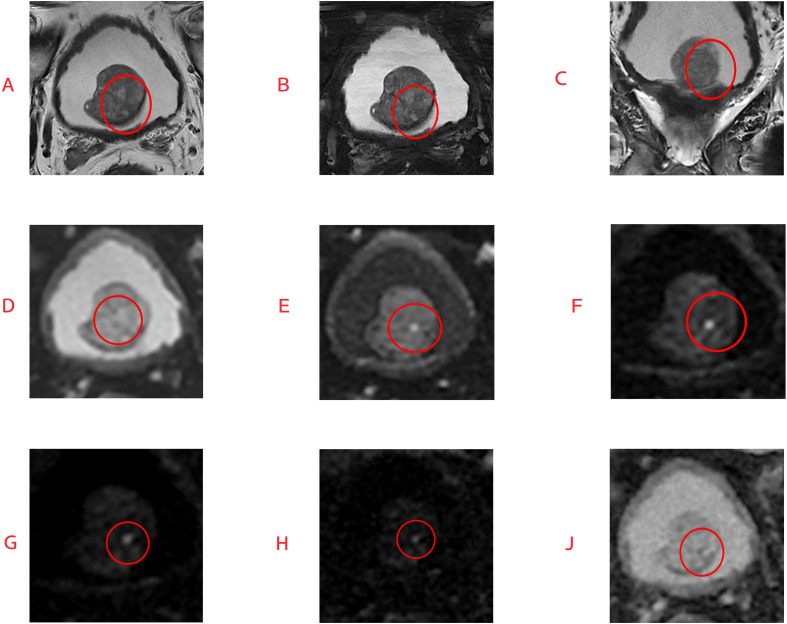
The patient is a 59-year-old male. Prostate MRI images reveal: blurred lesion margins on T2-weighted images (A–C); DWI sequences at b=0 (D) and b=800 (E) are also shown; however, on DWI sequences with b=1500 (F), b=2500 (G), and b=3000 (H), as well as the ADC map (J), the region (circled in the image) demonstrates distinct diffusion-restricted lesions. Final pathological diagnosis confirmed transitional zone prostate cancer with a Gleason score of 7.
